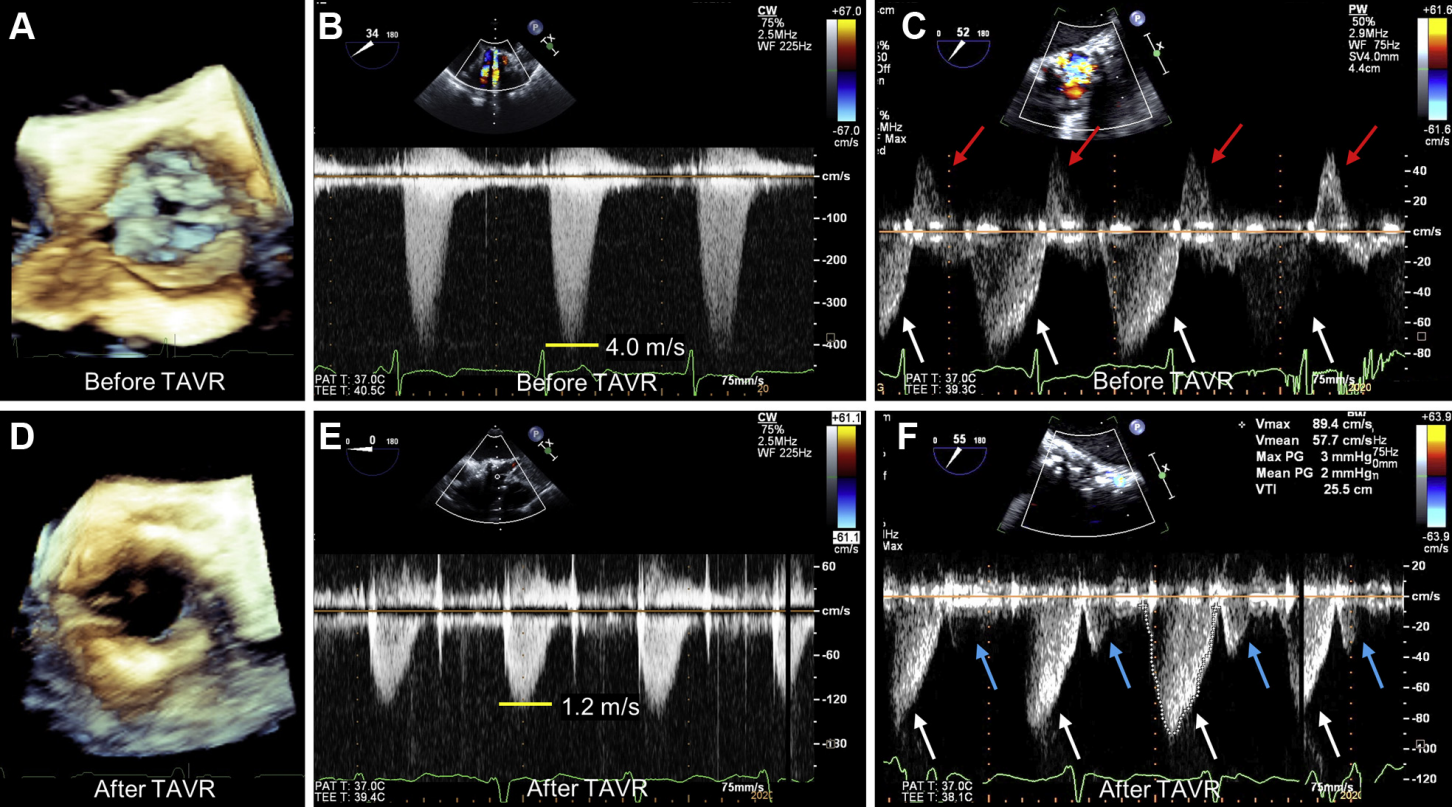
Transesophageal Echocardiography Before and After TAVR (A) Severely restricted aortic cusp motion. (B) Aortic valve peak velocity of 4.0 m/s before transcatheter aortic valve replacement (TAVR). (C) Coronary flow pattern before TAVR: reversed coronary flow during systole (red arrow) and forward coronary flow during diastole (white arrow). (D) Aortic valve complex after TAVR. (E) Aortic valve peak velocity of 1.2 m/s after TAVR. (F) Coronary flow pattern after TAVR: biphasic forward flow with small systolic (blue arrow) and large diastolic flow (white arrow).
